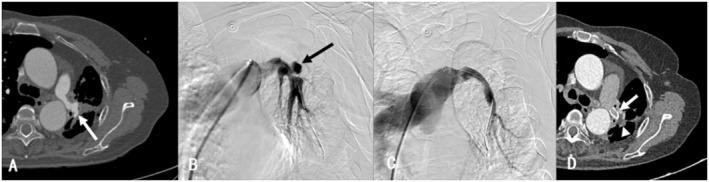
(A) Enhanced CTPA shows a saccular contrast agent filling shadow in the left main pulmonary artery. (B) Left main pulmonary artery angiography shows opacification of the pseudoaneurysm (black arrow). (C) Angiography after endovascular exclusion with covered stent shows no opacification of the pseudoaneurysm sac. (D) Chest enhanced CT at 1 year after surgery shows that the stent in the left main pulmonary artery is in good position with smooth blood flow and no opacification of the original pseudoaneurysm.
